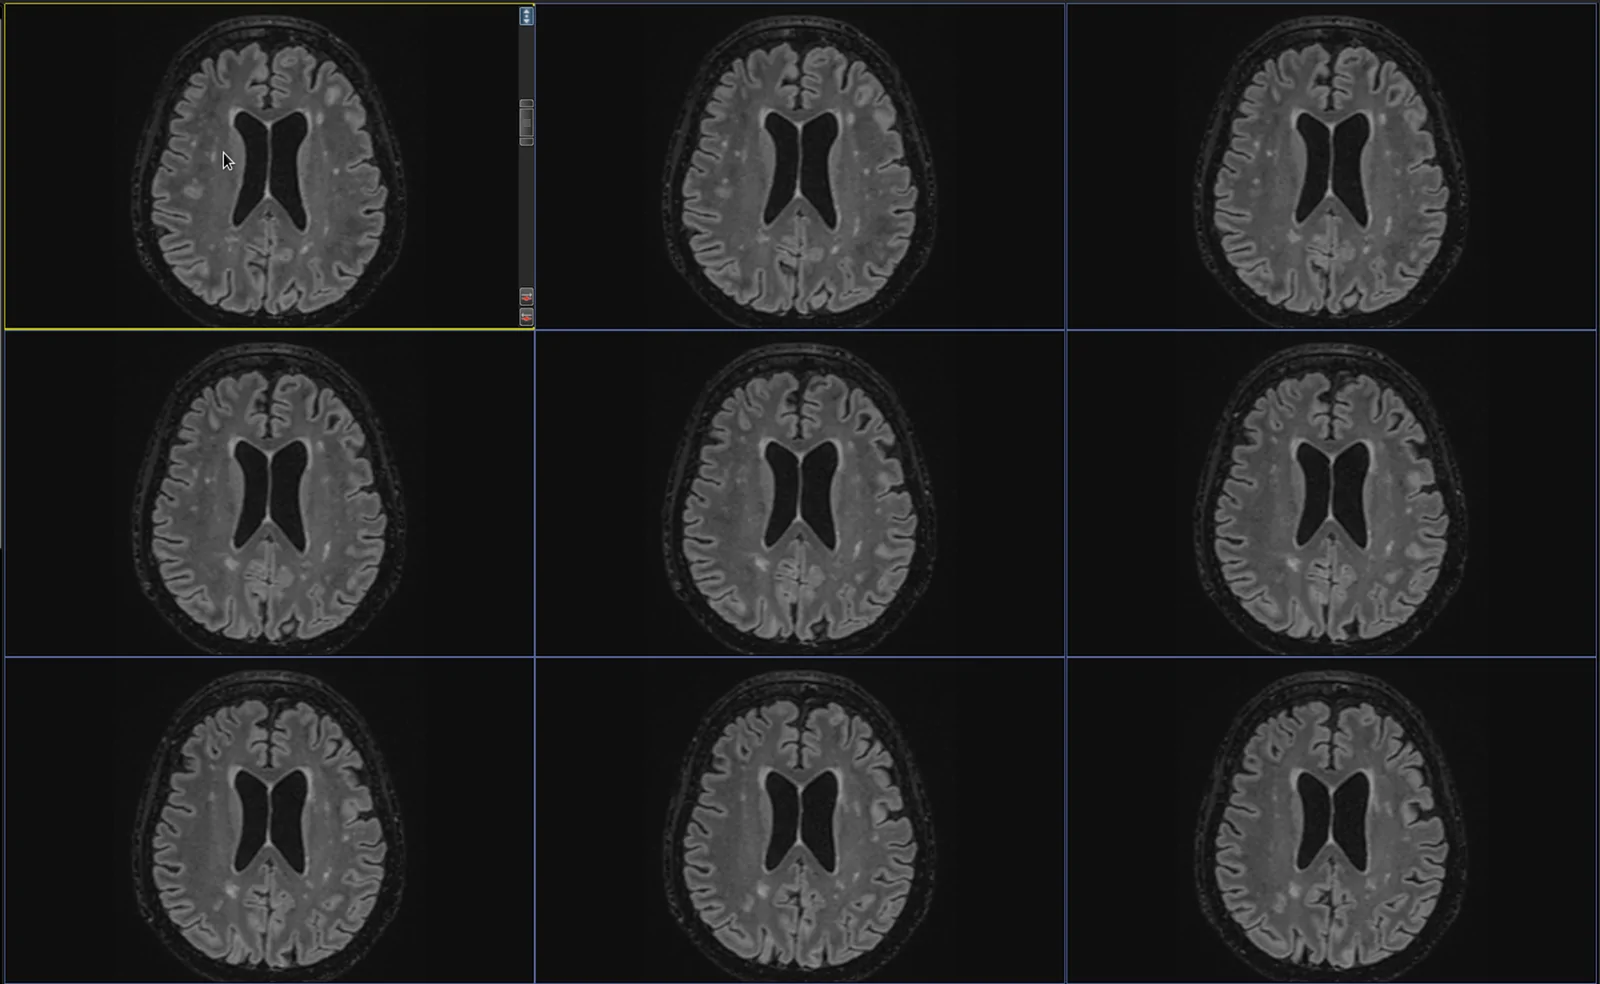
Brain magnetic resonance imaging (MRI). Axial T2-weighted/fluid-attenuated inversion recovery (FLAIR) sequences demonstrating multiple, partially confluent areas of hyperintensity in the subcortical, deep, and periventricular white matter, consistent with non-specific gliosis.
